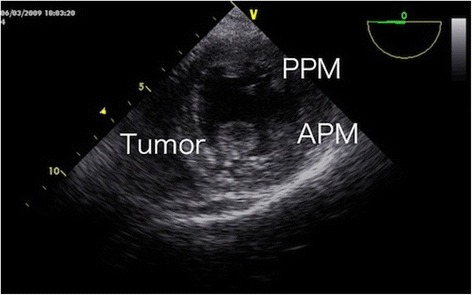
Intraoperative transesophageal echocardiography showing a round tumor originating from the anterolateral wall of the left ventricle. *APM*, anterior papillary muscle; *PPM*, posterior papillary muscle
